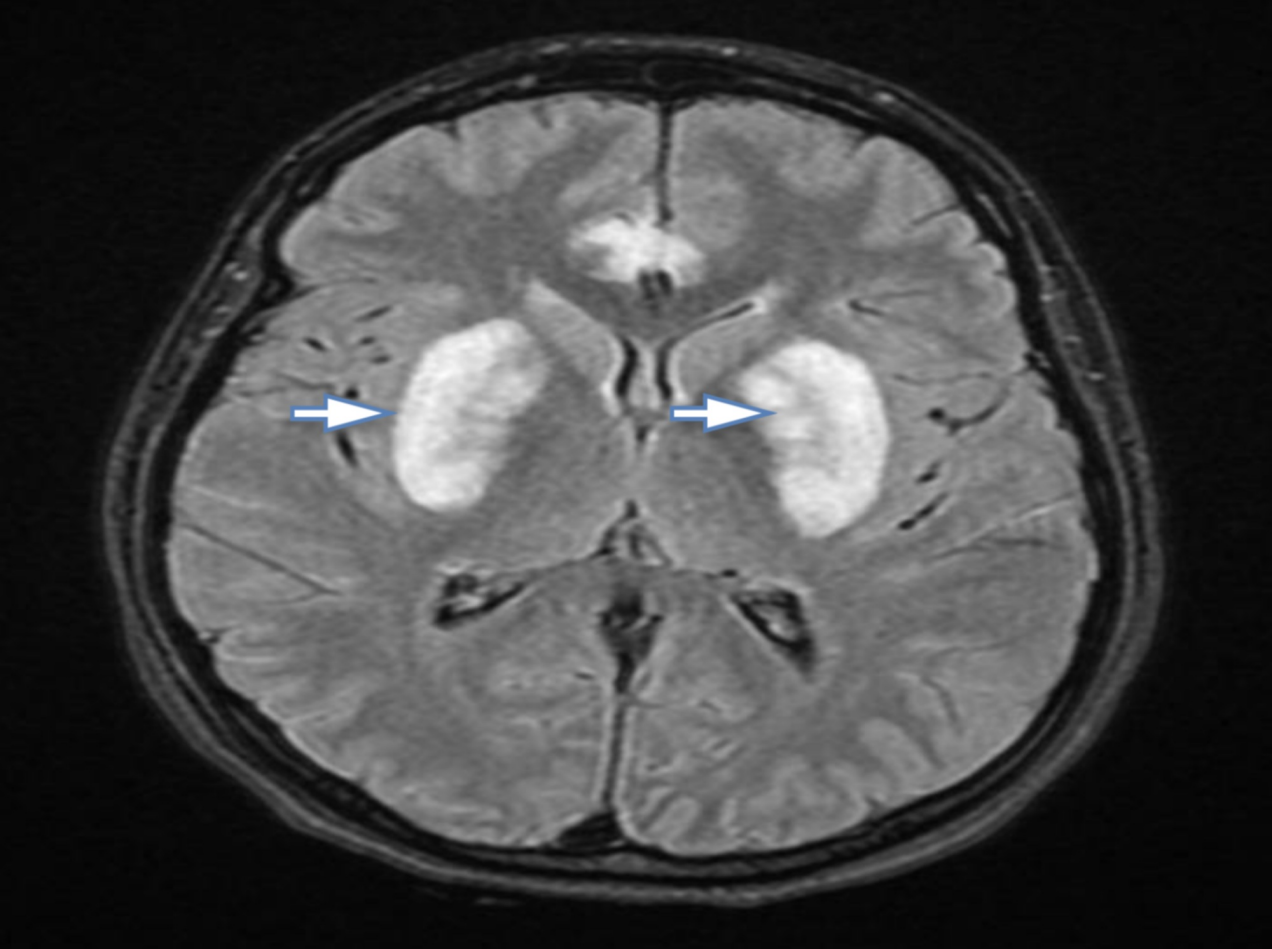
Cranial MRI showing multiple hyperintensities including basal ganglia
